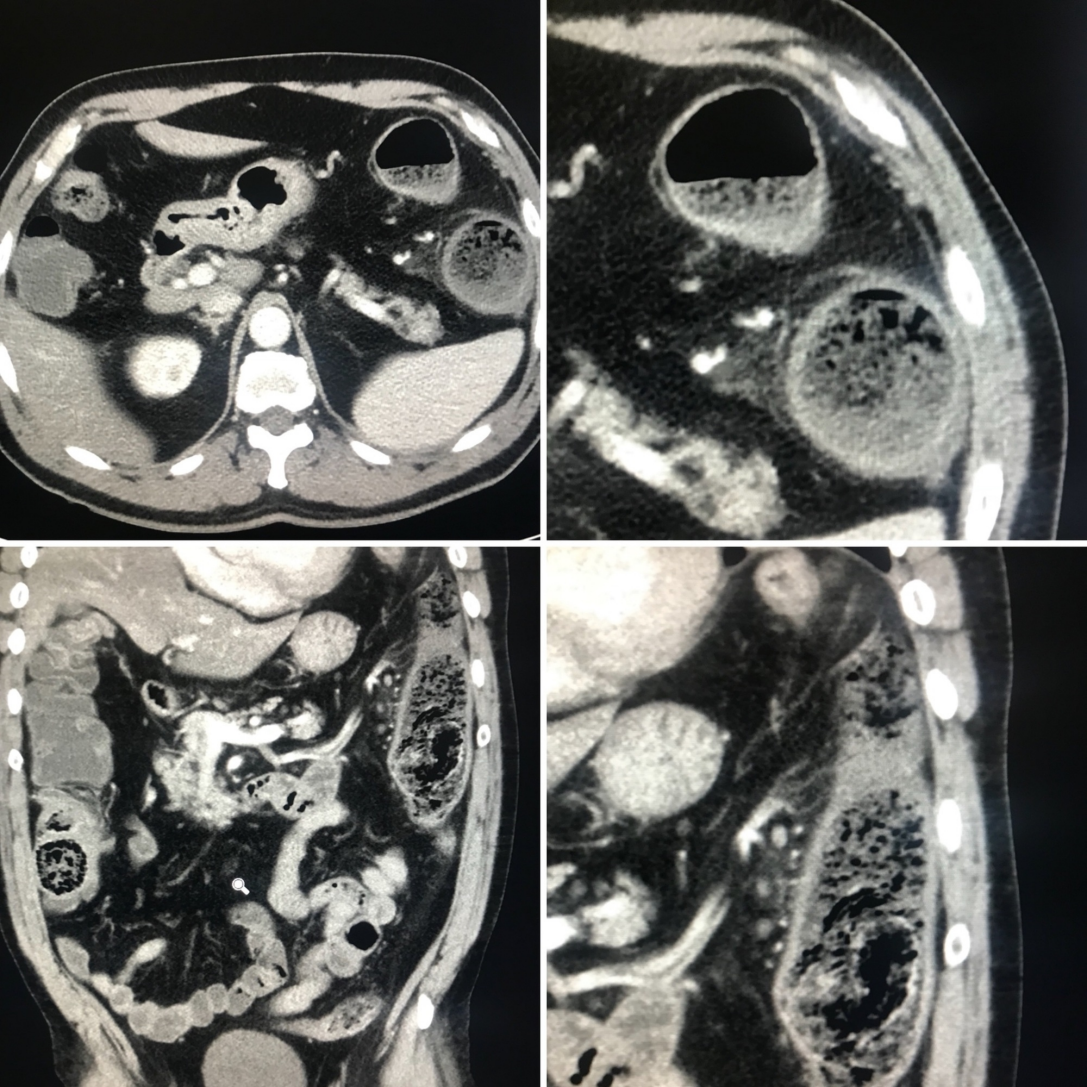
Focal colitis Axial (top) and coronal (bottom) views of the computed tomography scan of the abdomen with evidence of fecalith in the proximal and mid-descending colon associated with focal colonic wall thickening, pericolonic inflammatory stranding, and pericolonic fluid consistent with focal colitis
